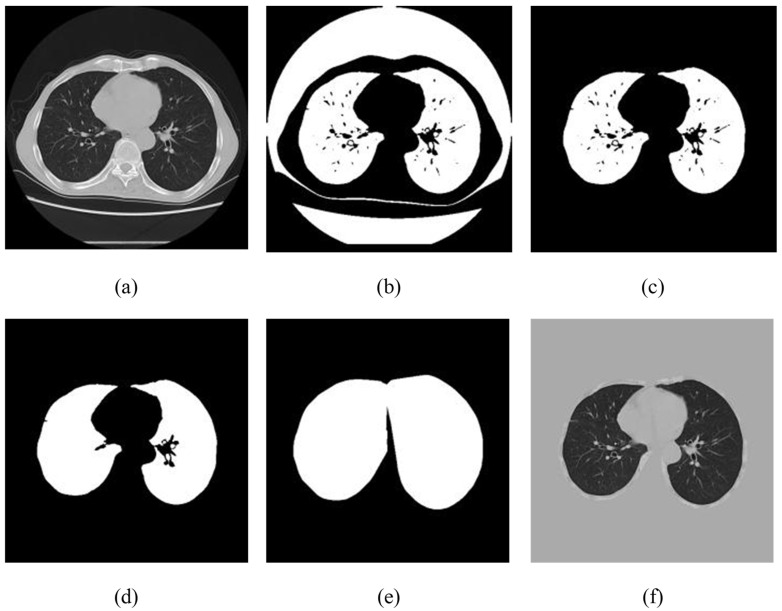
Resulting images of the preprocessing steps: (a) re-sampling of CT images with normalization; (b) CT image binarization with HU threshold ~600 estimated; (c) after the separation of unrelated areas; (d) after the application of erosion and dispersion operation; (e) mask construction on the image via convex hull operation; (f) fusing image of (a) and mask (e) to generate a valid pulmonary region.
